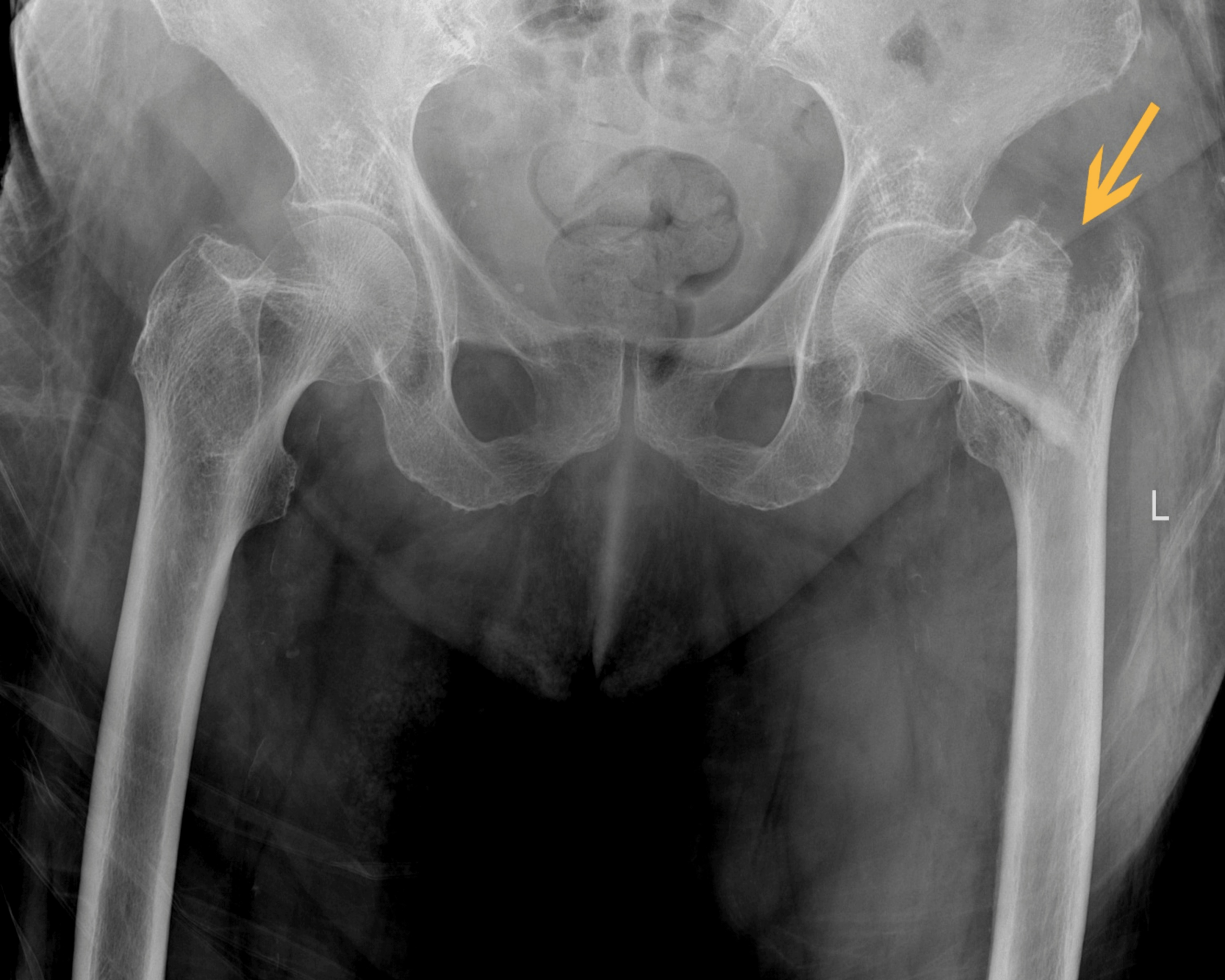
A pelvic radiography A yellow arrow showing an unstable trochanteric fracture of the left hip.
